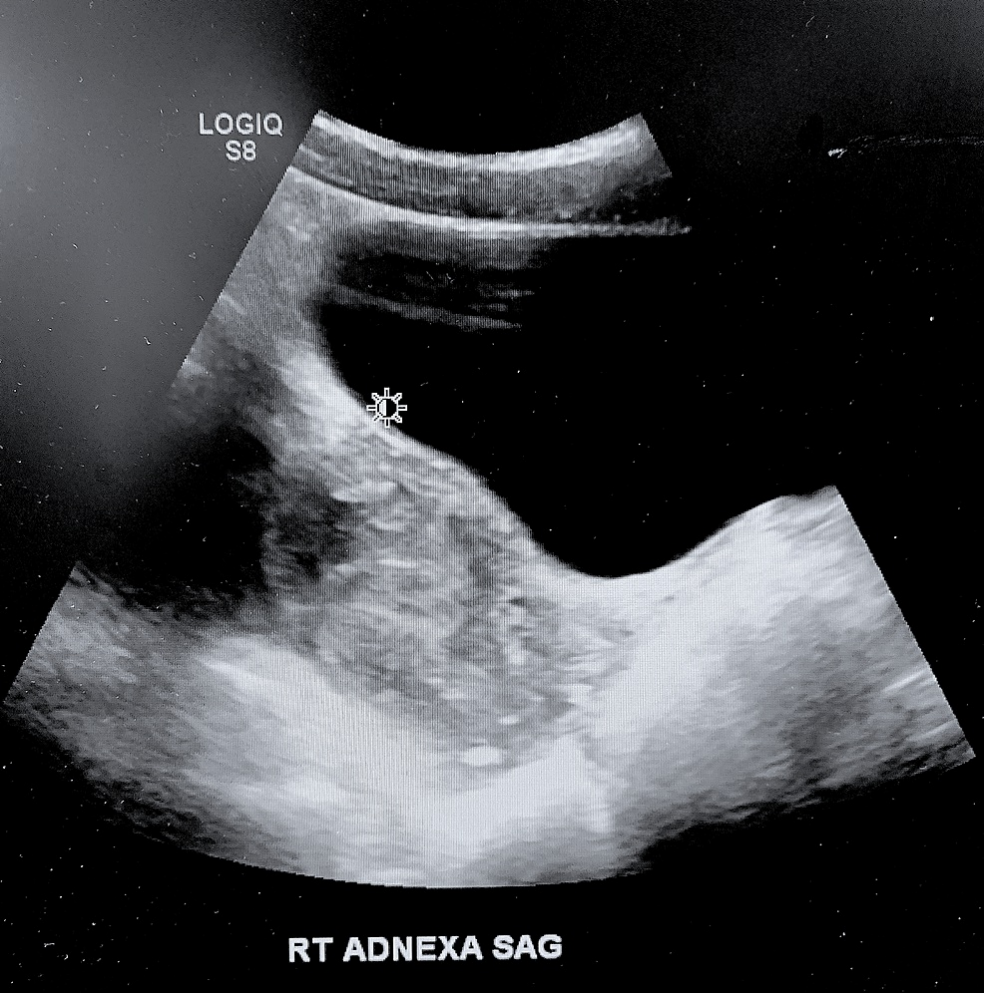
Pelvic ultrasound showing heterogenous mass adjacent to right ovary.
